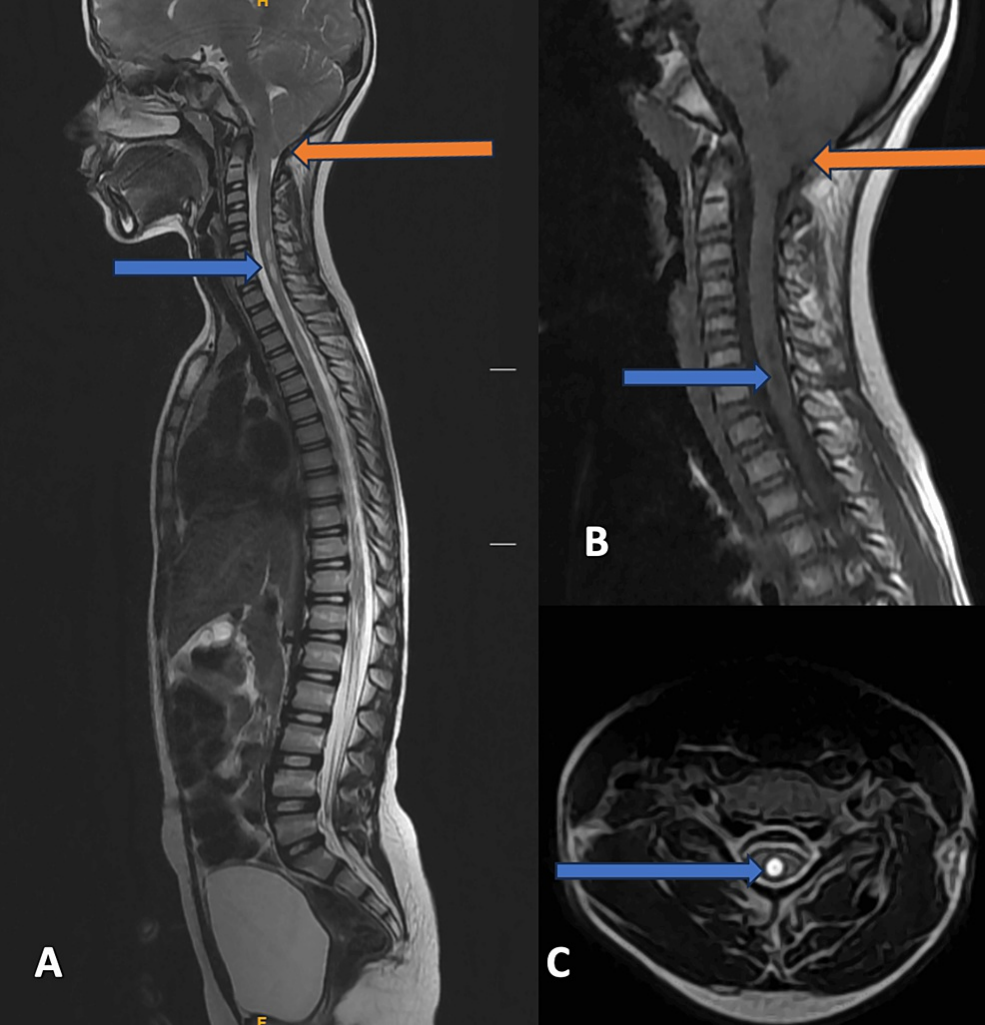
MRI of the cervical spine with whole-spine screening Magnetic resonance imaging (MRI) of the whole spine, mid-sagittal T2-weighted images, and mid-sagittal T1-weighted images of the cervical spine show tonsillar herniation (orange arrow) and hydrosyringomyelia (blue arrow) (A and B). The axial T2-weighted MRI image shows hydrosyringomyelia (blue arrow) (C).
